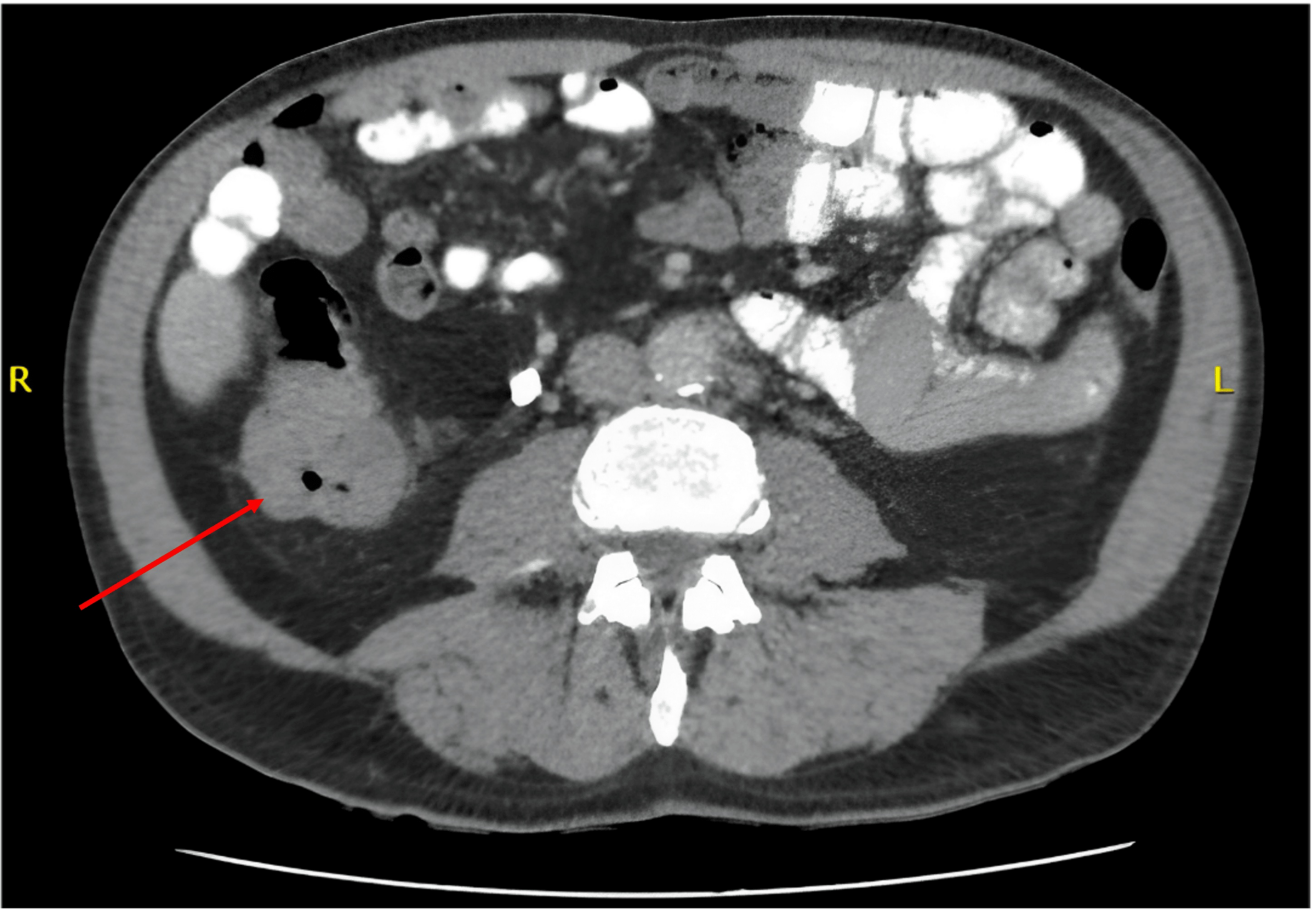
Axial view slice of contrast enhanced CT at the level of the hepatic flexure demonstrating a 6cm mass (red arrow) consistent with a primary colonic tumour
